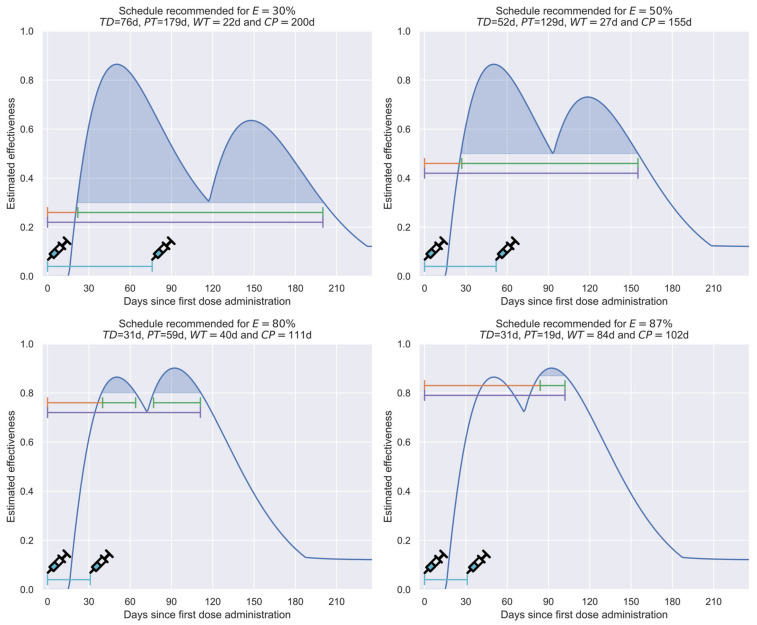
Comparison of the best schedules found in the optimization for 30%, 50%, 80%, and 87% minimum effectiveness values. TD, PT, WT, and CT are graphically included on each plot as blue, green, orange, and purple horizontal lines, respectively. The vaccination days are also graphically indicated with a syringe icon.
